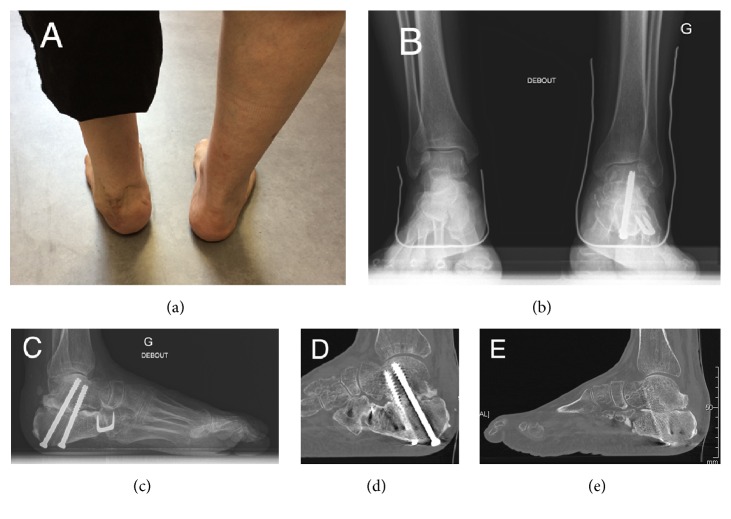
Latest follow-up assessment: Photograph shows a slight varus of the hindfoot (a). X-ray (b) and scan (c) assessment at 12-month follow-up with Meary incidence showing the residual varus of the hindfoot.
